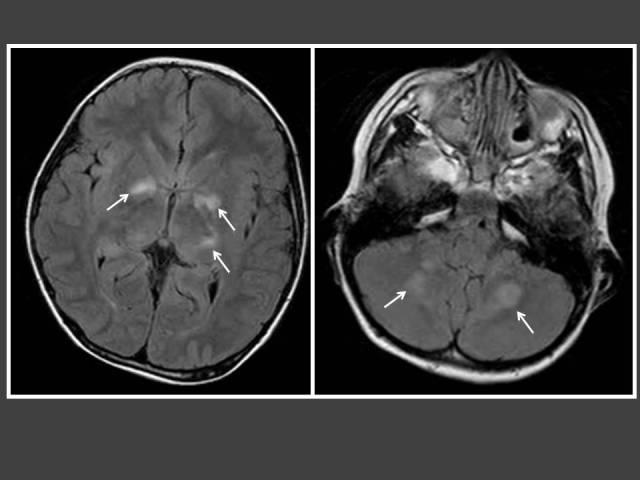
Seven year-old boy with neurofibromatosis Type 1 (NF-1) who presented with hirsutism. T2-FLAIR MRI of the brain shows multiple foci of high signal intensity in the basal ganglia and cerebelli (arrows) consistent with myelin vacuolation.
